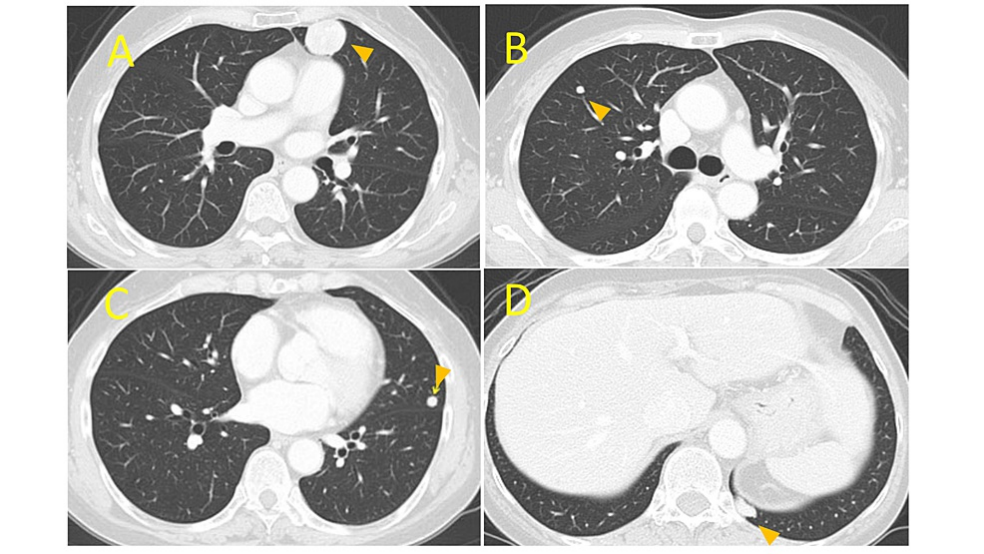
Multiple lung metastases in computed tomography Different-sized multiple lung metastases were indicated bilaterally. (A) Left upper lung S3 (arrowhead): metastatic tumor with 5 cm diameter. (B) Left lower lung S10 (arrowhead): metastatic tumor with 1 cm diameter. (C) Right upper lung S3 (arrowhead): metastatic tumor with 1 cm diameter. (D) Left lingula lung* S5* (arrowhead): metastatic tumor with 2 cm diameter.
